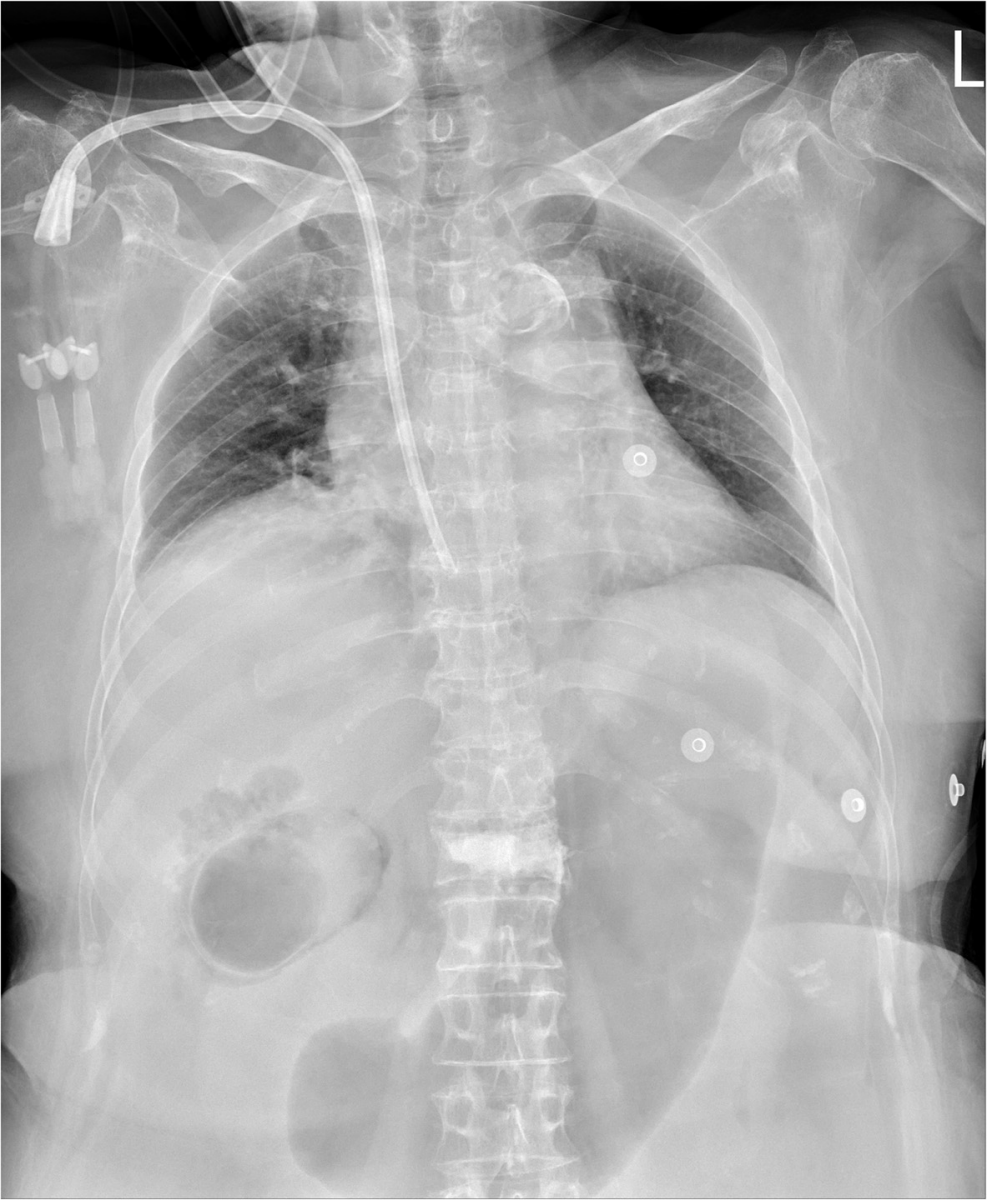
Plain-film radiography showing air in the lumen and wall of the enlarged gallbladder of a 64-year-old woman with abdomen pain and shock while undergoing hemodialysis (arrows)
